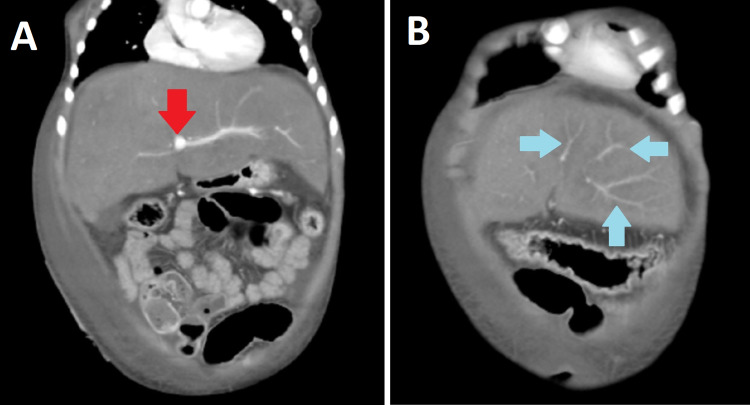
Axial contrast triphasic CT scan of abdomen. Image A shows occlusion of the portal vein (red arrow). Image B shows formation of collaterals in liver (blue arrows). CT, computed tomography
